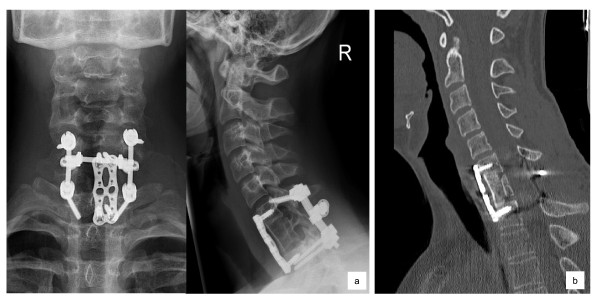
a) The conventional X-Rays at 12 months follow-up show the correct alignment of the cervical spine with the intact bone graft and spondylodesis implants. b) The computertomography at 12 months follow-up shows good bony consolidation and the unaltered and correct alignment of the cervical spine.
